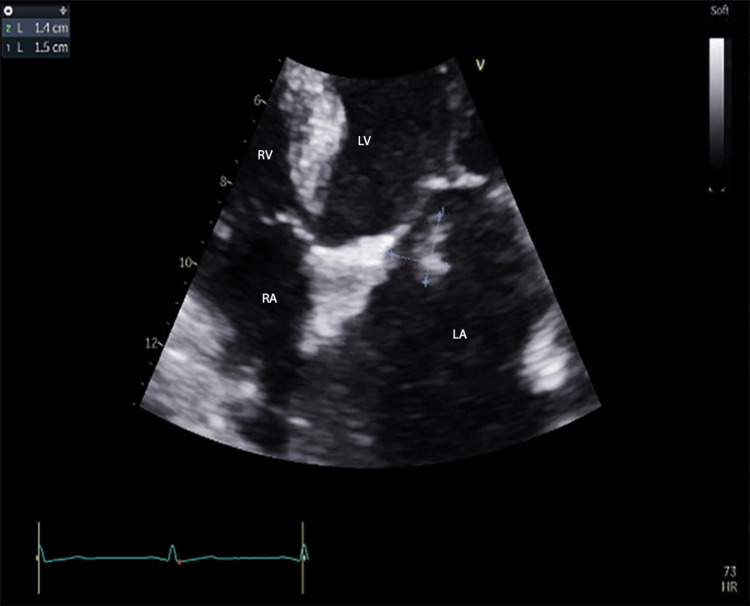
TTE results from the patient’s hospital admission with measurement of the echogenic mass in the atrial aspect of the anterior mitral leaflet. TTE: transthoracic echocardiogram
